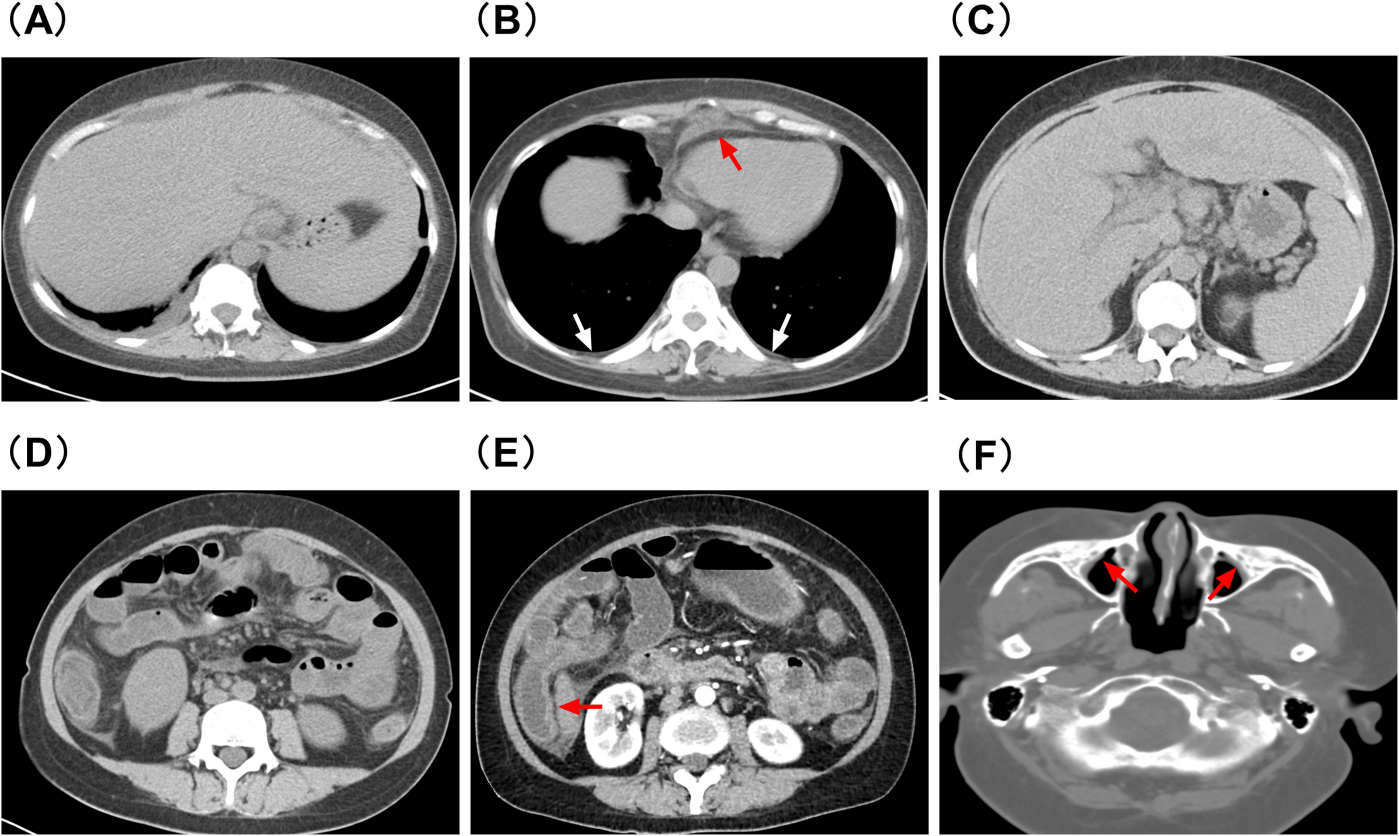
(A–D, F) are plain CT scans, and (E) is contrast-enhanced CT. (A) Bilateral pleural effusion (B) Pleural thickening (white arrow) and pericardial effusion (red arrow) (C) Hepatomegaly, splenomegaly, and multiple enlarged lymph nodes (D) Multiple abdominal exudates with blurred fat spaces and flocculent high-density shadows (E) Thickened ascending colon wall (red arrow) and exudation around the transverse colon (F) Bilateral maxillary sinus mucosal thickening (red arrow).
